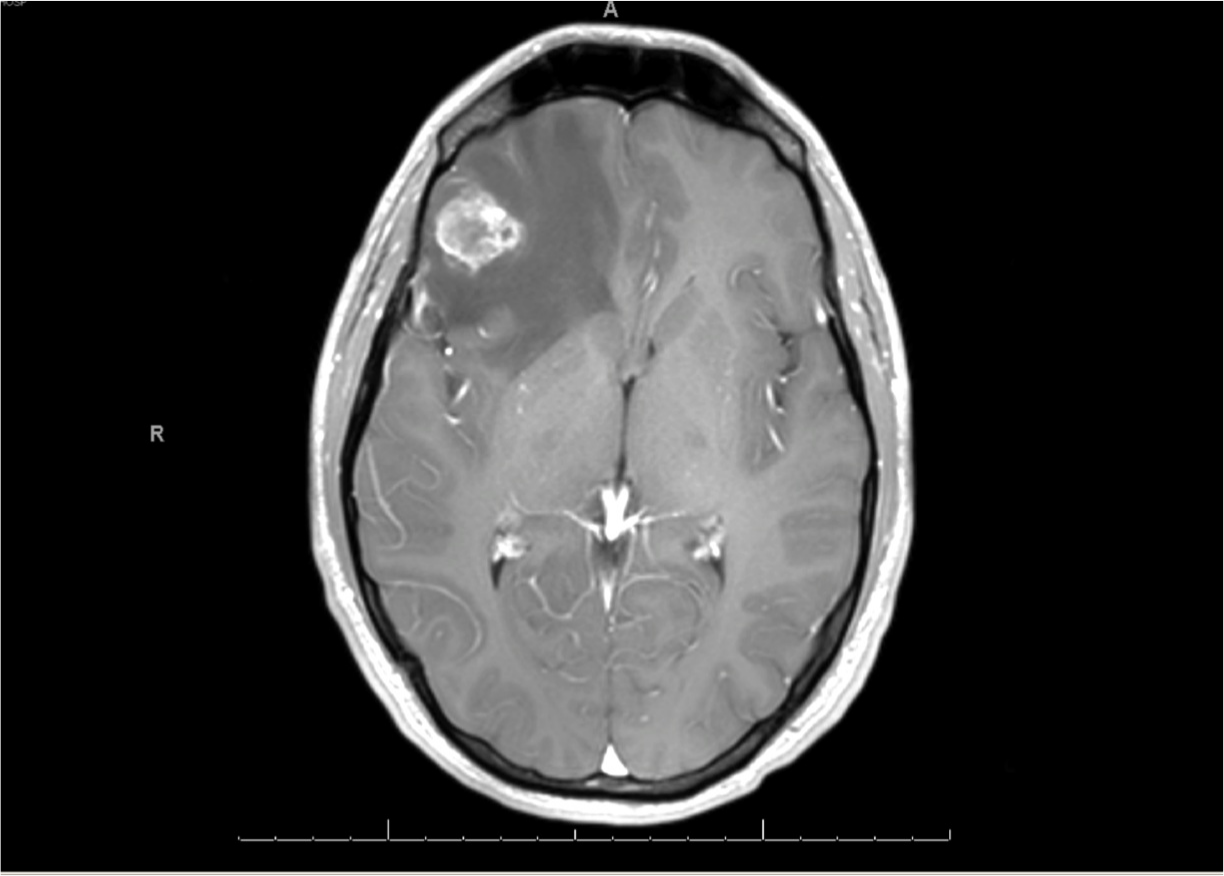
Initial Brain MRI Single intra-axial heterogeneously enhancing mass in the inferior aspect of the right frontal lobe.
